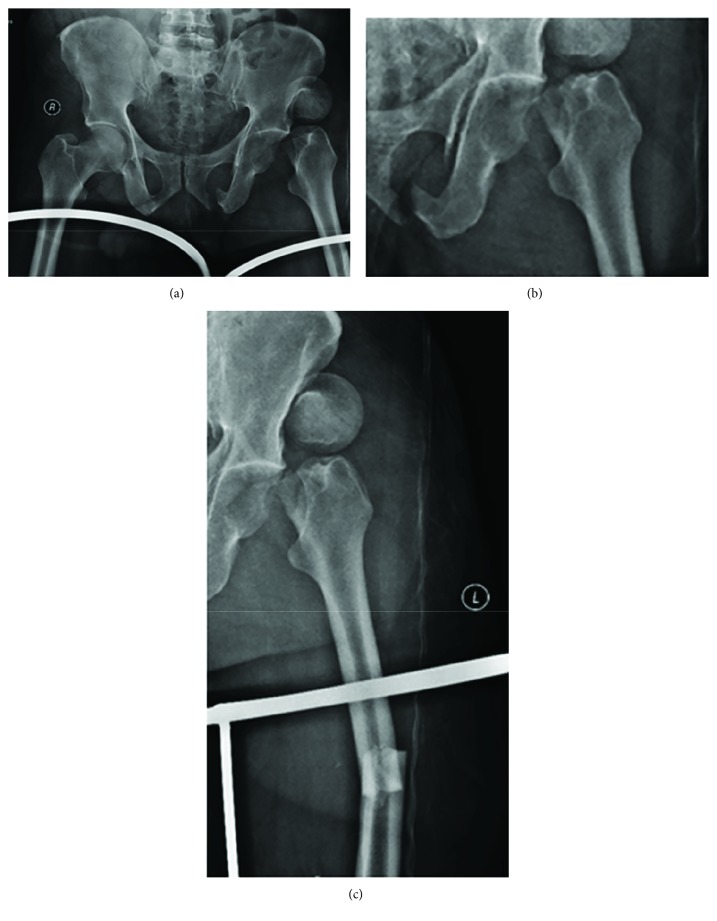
Radiographs of the pelvis (a), showing a posterior wall acetabulum fracture (b) with a displaced femoral head and a concomitant femur shaft fracture (c).
